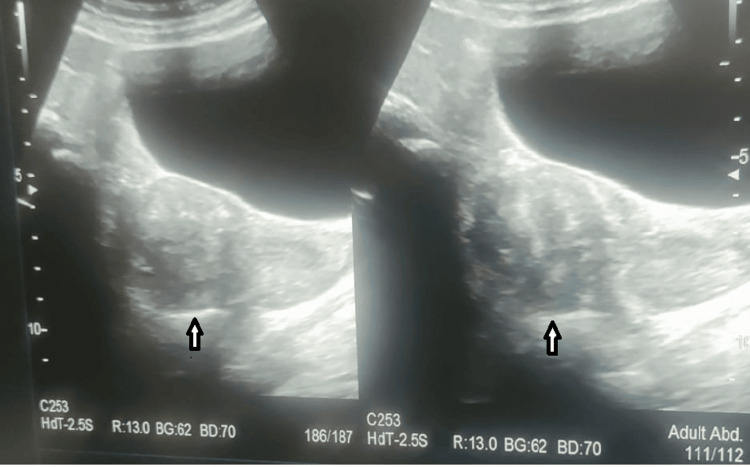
Ultrasound suggestive of invasive mole The arrow depicts a well-defined heterogeneous structure with cystic areas within the endometrium.
